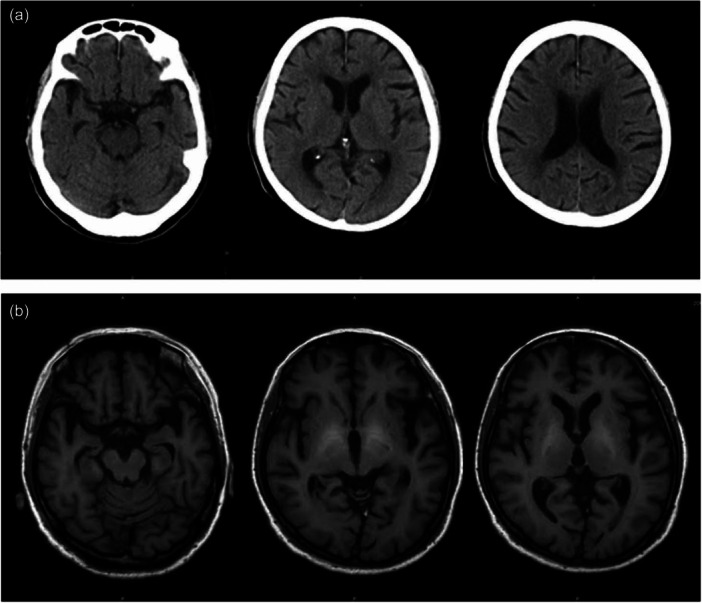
Morphologic brain imaging. Head Computed Tomography was unremarkable at admission to the psychiatric hospital (a). T1‐weighted brain magnetic resonance imaging revealed hyperintensity in the bilateral basal ganglia when overt hepatic encephalopathy was diagnosed (b).
